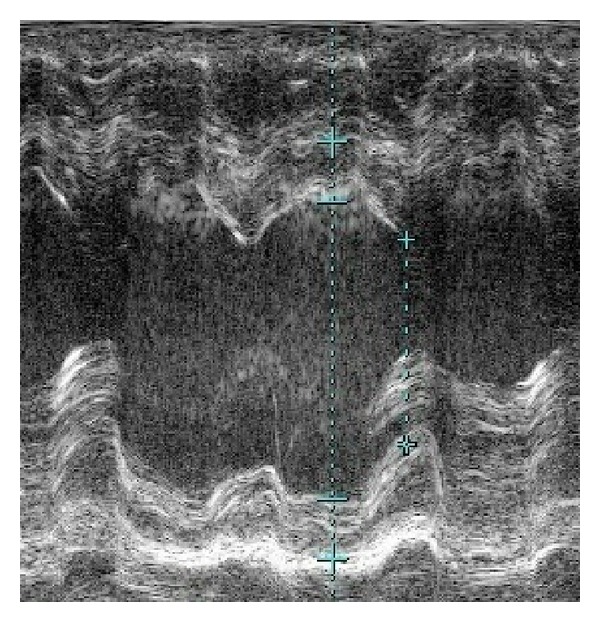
Echocardiography shows preserved left ventricular ejection fraction of 58% without regional wall motion abnormalities.
